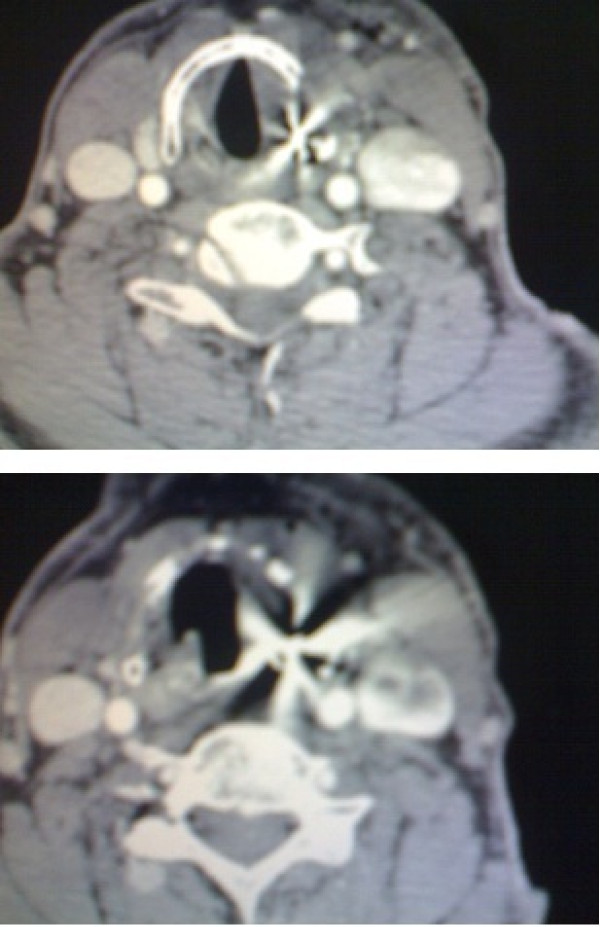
Computer tomography scan of the neck. Computed tomography showed the depth of penetration but was not very accurate.
